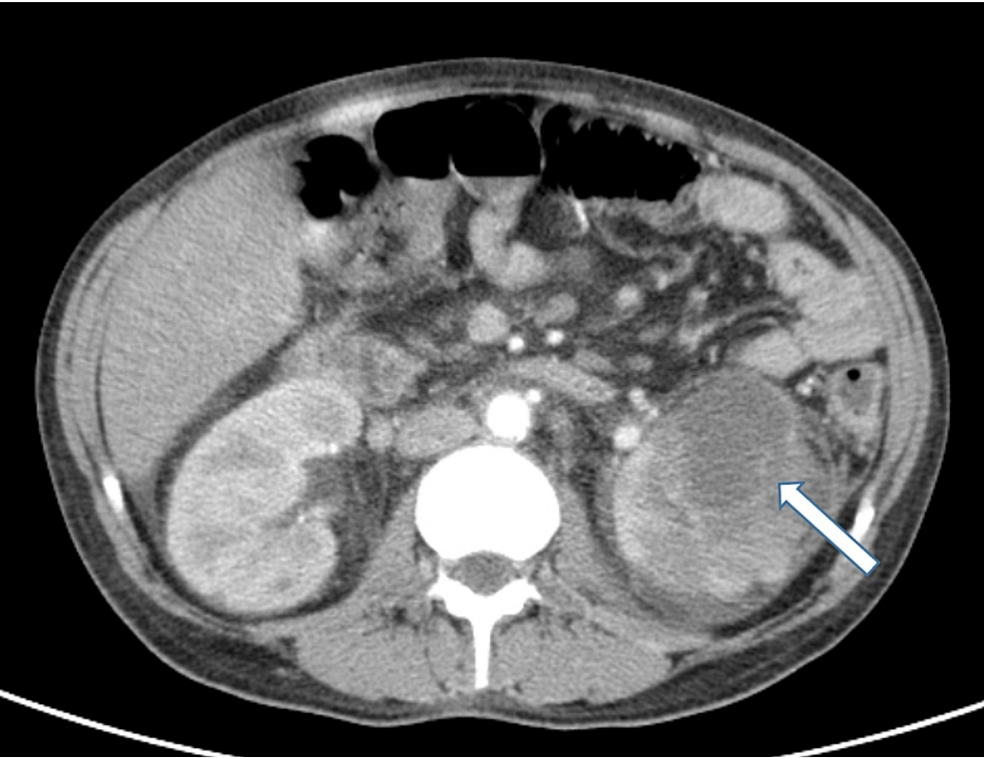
Abdominopelvic CT scan showing the presence of renal abscesses, 7 cm on the left (white arrow) and 3 cm on the right CT: computed tomography
